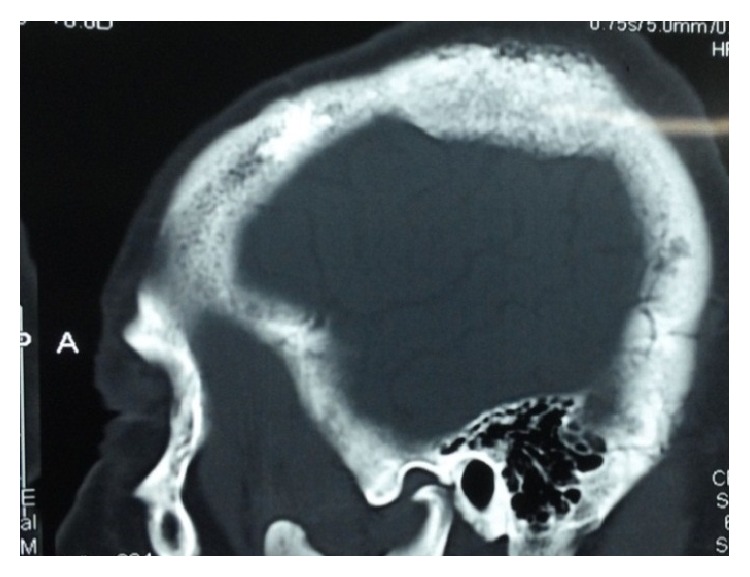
CT of the brain 12 months after androgen deprivation showing decrease in size of the parietal mass and bony exostosis.
